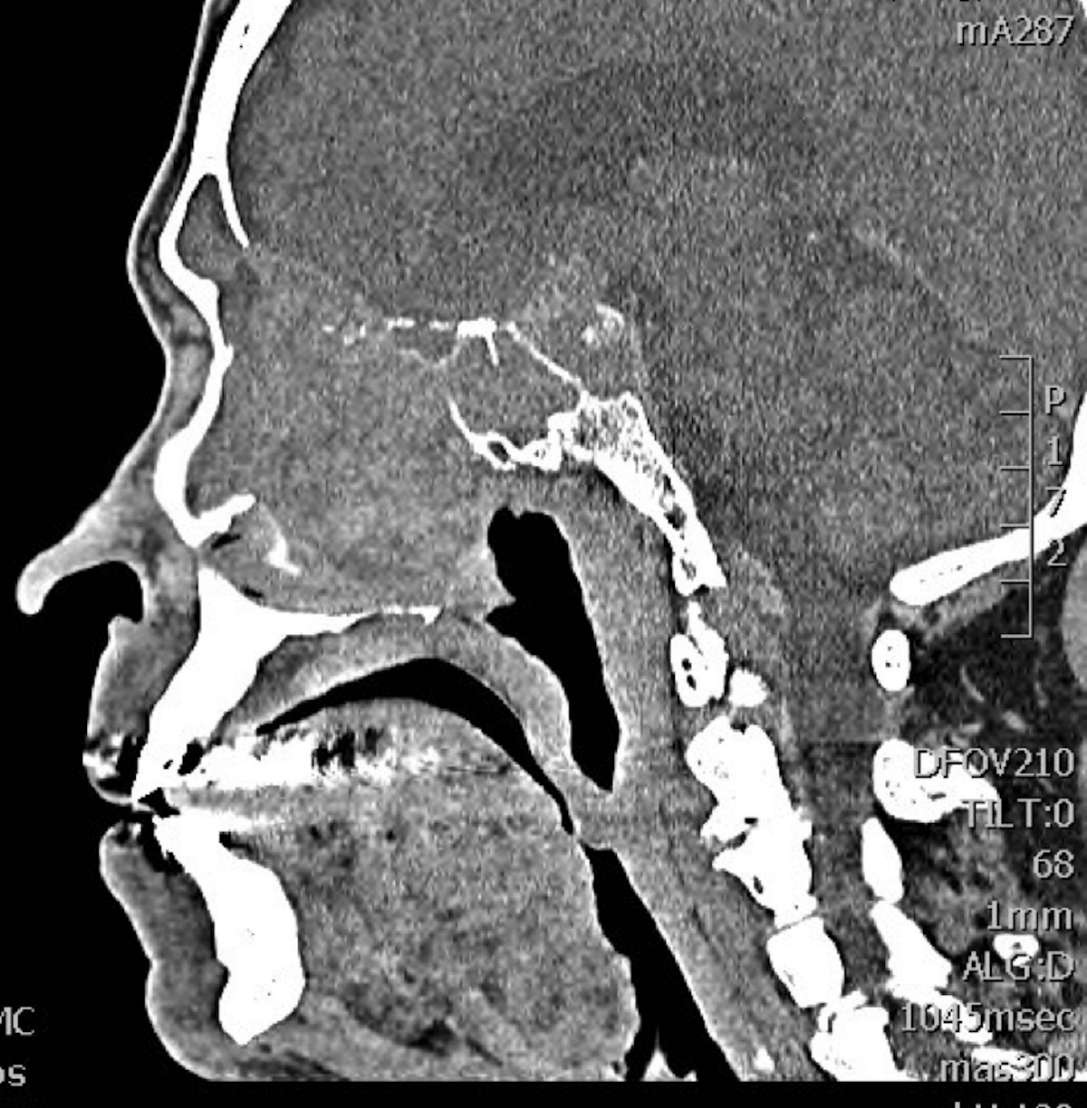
Contrast-enhanced computed tomography scan Sagittal cut showing the lesion involving the ethmoid sinuses, invading the nasal cavity and septum, the frontal and sphenoid sinuses, with base of skull erosion and intracranial extension.
